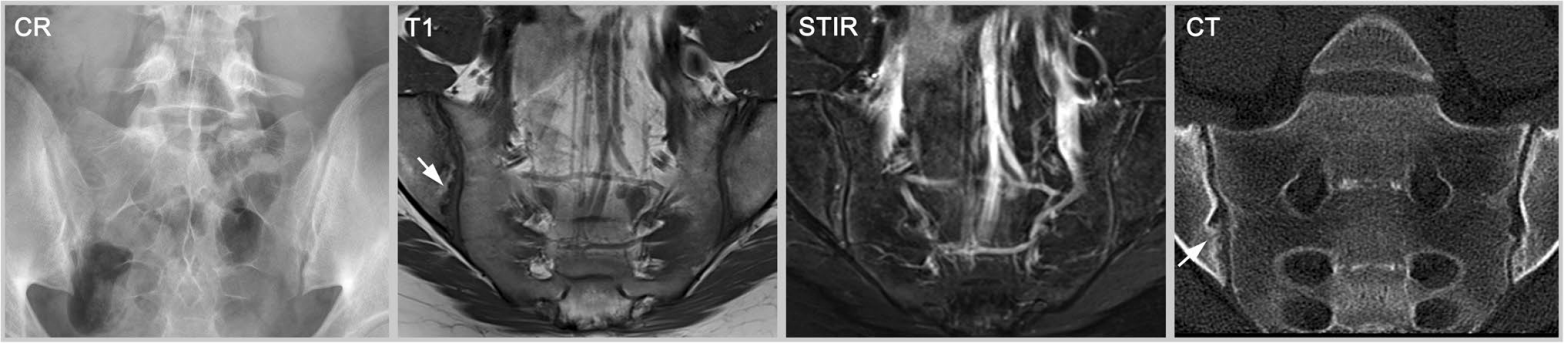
Early axSpA without active inflammation. This 24-year-old male patient has negative radiography but unequivocal erosions in MRI-T1 and CT (arrows). However, he shows no osteitis on STIR. While he does not fulfill the definition of a positive imaging test (radiography is negative and MRI shows no active inflammation), the erosions still support a clinical diagnosis of axSpA
